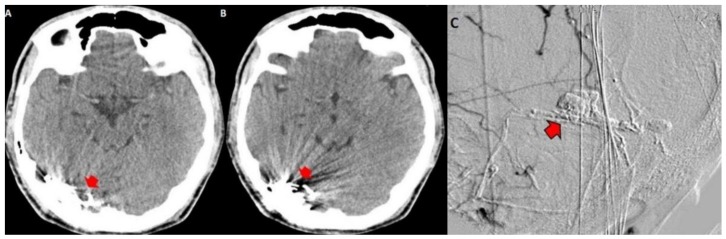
Contrasted CT showing embolized dural arteriovenous fistula with Onyx by endovascular procedure. This material produces artifacts with image distortion in the right occipital region (red arrow heads in (A,B)). The angiography after the therapeutic procedure of the fistula shows that it was successfully embolized, so it is not contrasted (red arrow with black outline in (C)).
